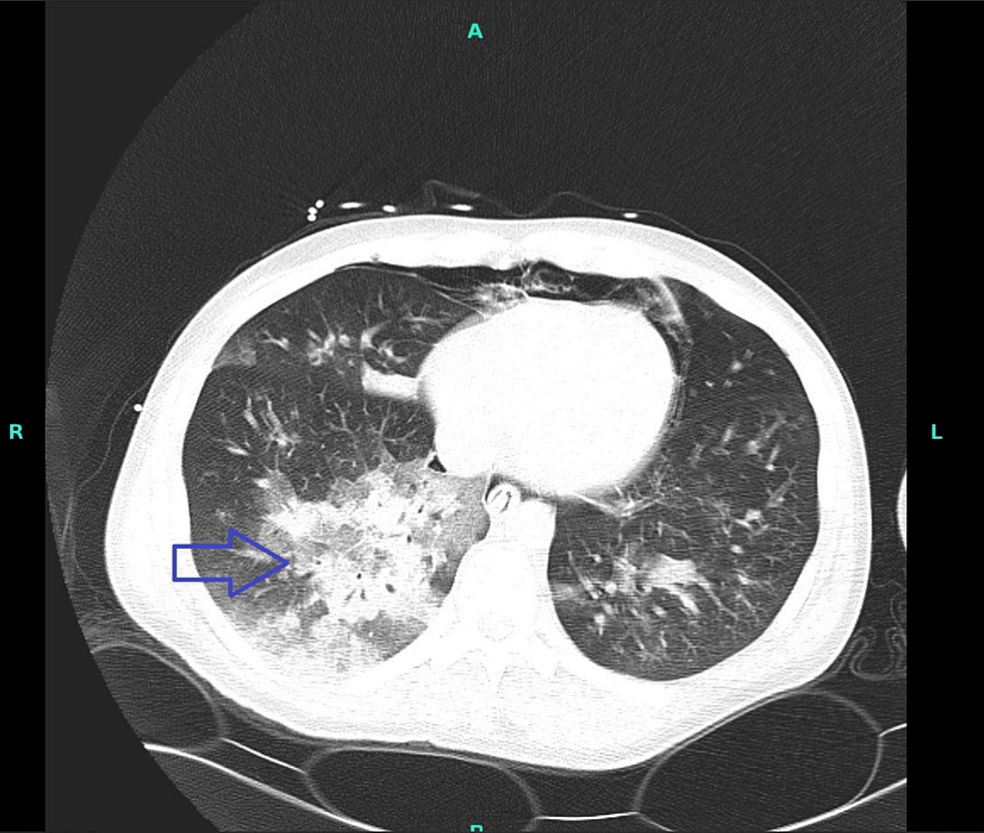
CT chest without intravenous contrast showing extensive bilateral centrilobular ground glass and consolidative airspace opacities throughout the lungs, bilaterally more pronounced in the right lower lobe (blue arrow)
